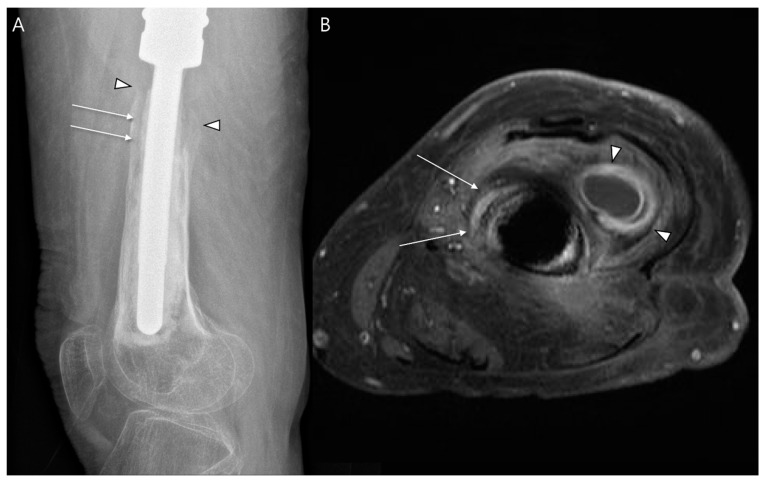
Periprosthetic infection following limb-salvage surgery for osteosarcoma performed 15 years ago. (A) Postoperative plain radiograph of the distal femur shows osteolysis surrounding the femoral stem of an endoprosthesis, measuring more than 2 mm (arrows), with blurred cortical remodeling (arrowheads). (B) Axial T1-weighted enhanced MR image shows periosteal enhancement (arrows) and soft tissue abnormalities with abscess formation (arrowheads), indicating periprosthetic infection rather than implant loosening.
